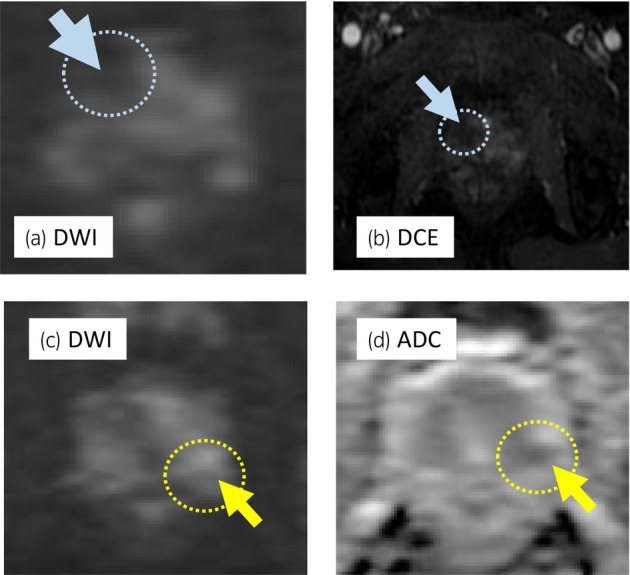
Surveillance MRI at 4 months after FT. The treated targeted lesion of focal therapy was normalized in DWI, and no enhancement in DCE (blue arrow with circle in a, b). MRI at 4 months after focal therapy revealed a new lesion of PIRADS‐category 4 in the left PZ (yellow arrow and circle in c, d).
